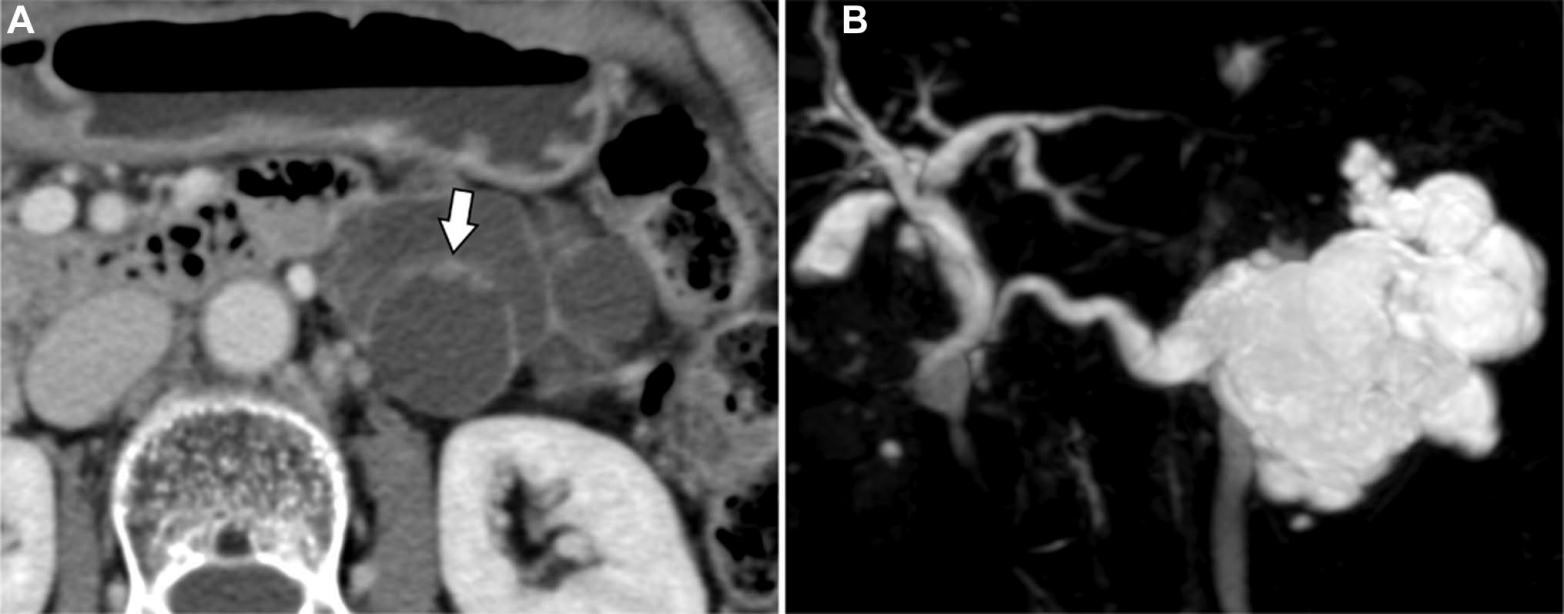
Case of an 80-year-old woman with a cystic mass in the pancreatic tail found on abdominal ultrasonography during a health checkup (Case 4). A well-demarcated multilocular cystic lesion with a small mural nodule is found in the tail of the pancreas on contrast-enhanced computed tomography (a: arrow). The solid portion shows a stronger contrast enhancement than the pancreas in the portal phase of contrast-enhanced computed tomography. Magnetic resonance cholangiopancreatography confirms the mass as a mixed type with prominent pancreatic duct dilatation downstream of the mass (b).
